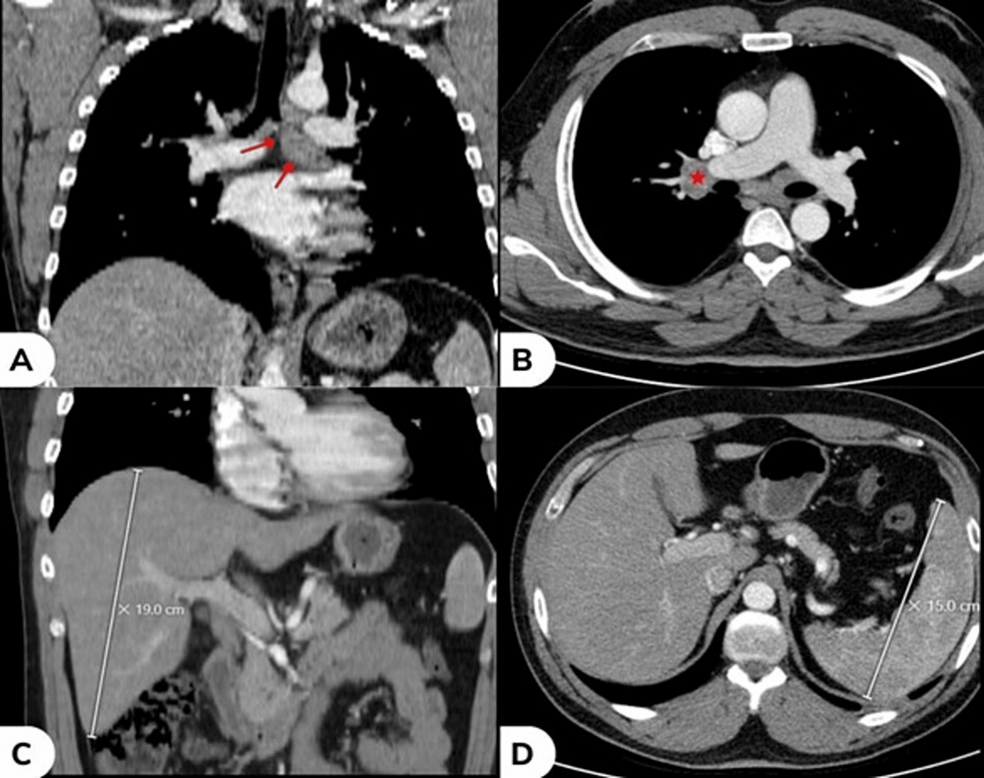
(A) Coronal view CT showing subcarinal lymphadenopathy (red arrows); (B) Axial view CT showing right hilar lymphadenopathy (red star); (C) Coronal view CT showing enlargement of the liver measuring 19.0 cm (white meter); (D) Axial view CT showing enlargement of the spleen measuring 15.0 cm (white meter)
